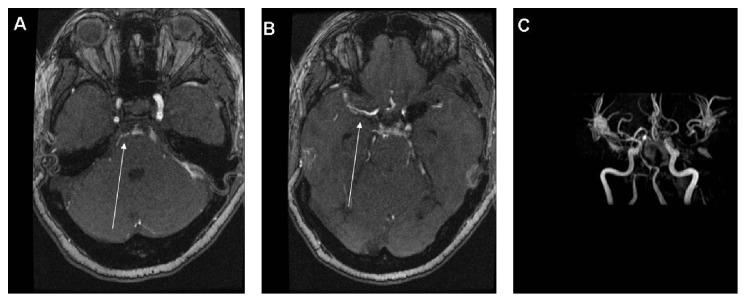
Postbleed day 5 MRI with TOF angiography. MRI = magnetic resonance imaging and TOF = time of flight. (A) Axial TOF sequence at the level of the pons displaying severe basilar artery spasm (white arrow). (B) Axial TOF sequence at the level of the mesencephalon displaying severe right middle cerebral artery vasospasm (white arrow). (C) Three-dimensional TOF reconstruction of the circle of Willis displaying severe spasm of the basilar artery trunk and the middle cerebral arteries bilaterally. The vasospasm is severe enough to prevent TOF signal from identifying the distal basilar and the left proximal middle cerebral artery.
